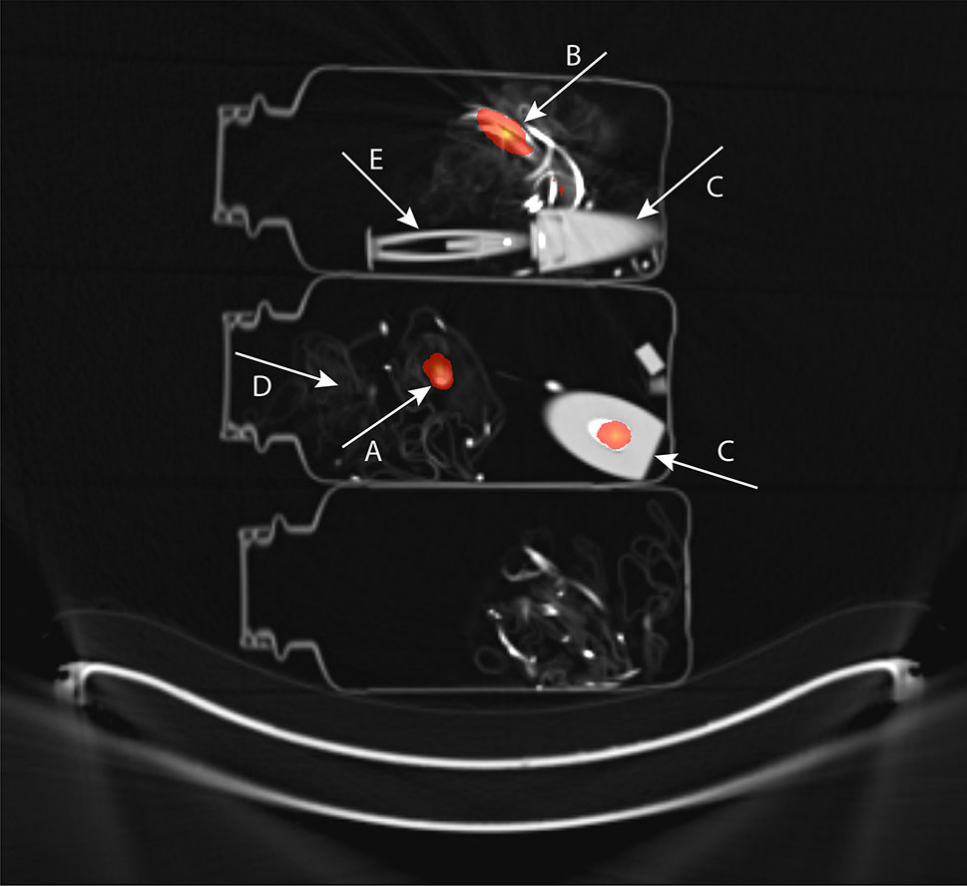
Combined images of PET and CT of three of the waste containers after radioembolization with glass microspheres showing that residual activity was found in the microcatheter connector (A), tubing (B) and V-vial (C). Other items that can be distinguished are the surgical cloth (D) and the plunger (E). ^89^Zr was used as an alternative isotope for acquisition and reconstruction (as ^90^Y as acquisition isotope was not available at that time). Activity recovery was corrected for the different half-life and positron branching ratio between ^89^Zr and ^90^Y
